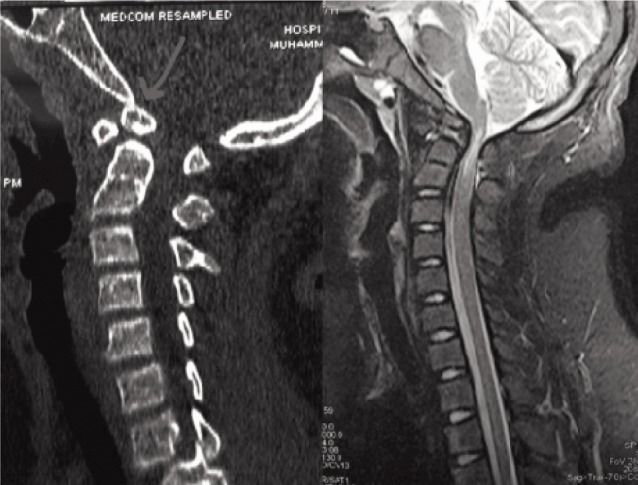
CT scan showing dystrophic form of os odontoideum. MRI showing the cervico-medullary cord compromise.
